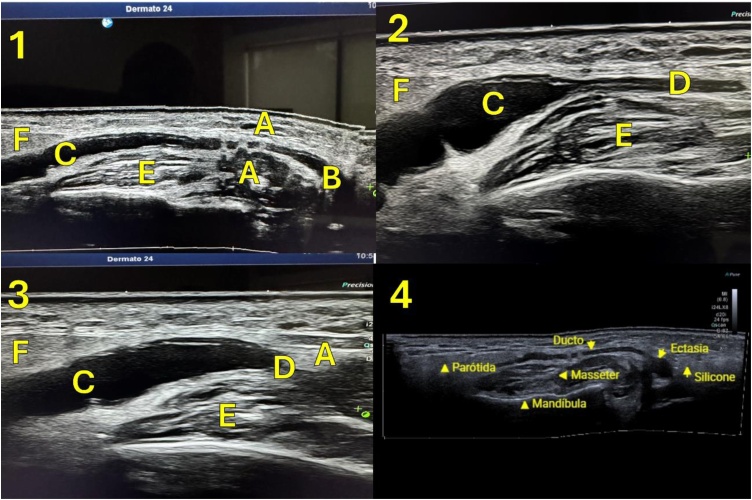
Dermatological ultrasound with stenosis and ectasia of the salivary duct due to compression by filler (silicone). (A) Silicone filler. (B) Duct. (C) Duct ectasia. (D) Duct stenosis. (E) Masseter. (F) Parotid.
